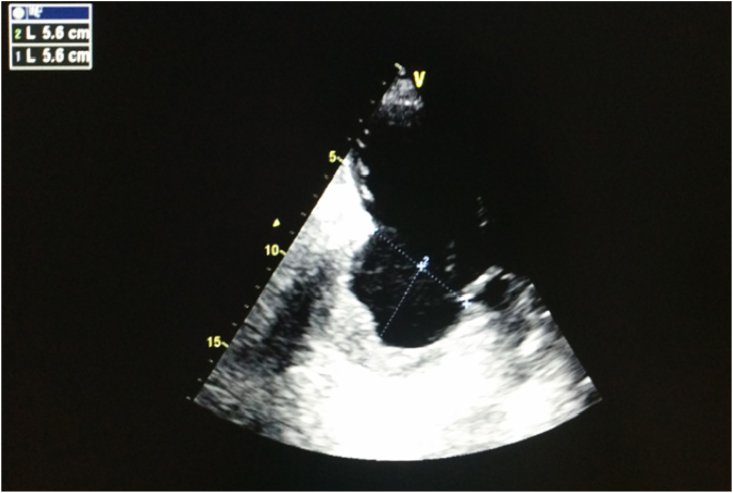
Echocardiographic image showing a chronic, thrombosed aneurysm of left ventricule inferolateral wall.
